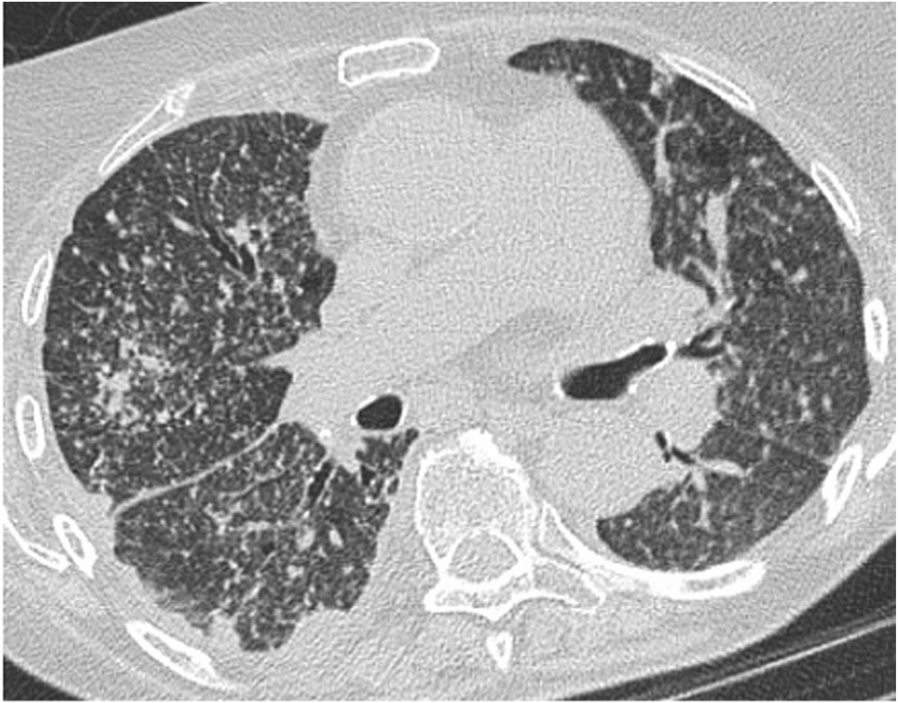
High-resolution computed tomography showing mildly enlarged lymph nodes, mostly right-sided pleural lesions, thickening of the fissures and interlobular septa, and bilateral diffuse micronodular opacities (miliary pattern).
